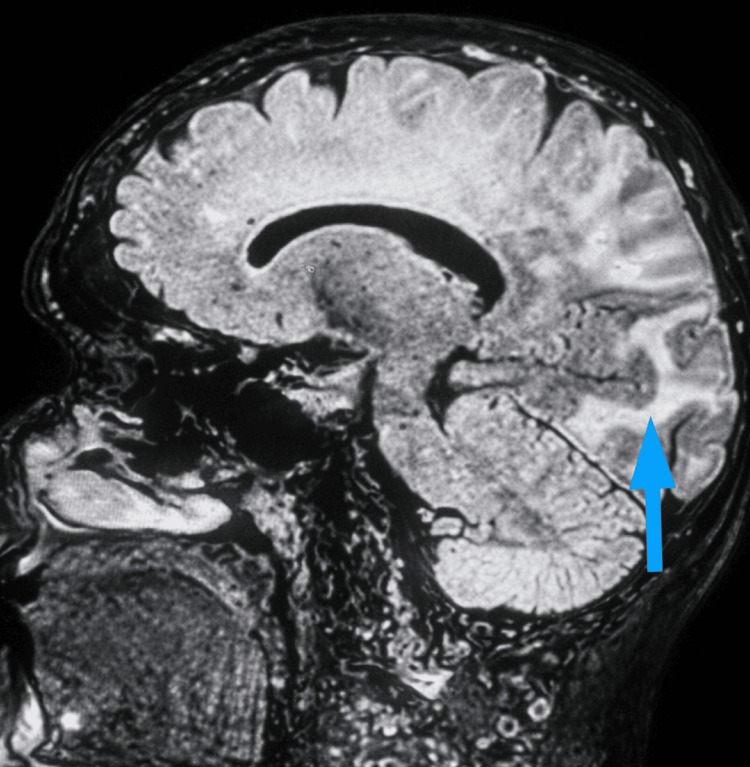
Sagittal fluid-attenuated inversion recovery (FLAIR) sequence demonstrating diffuse supratentorial white matter hyperintensities, most prominent in the parieto-occipital regions (blue arrow).
